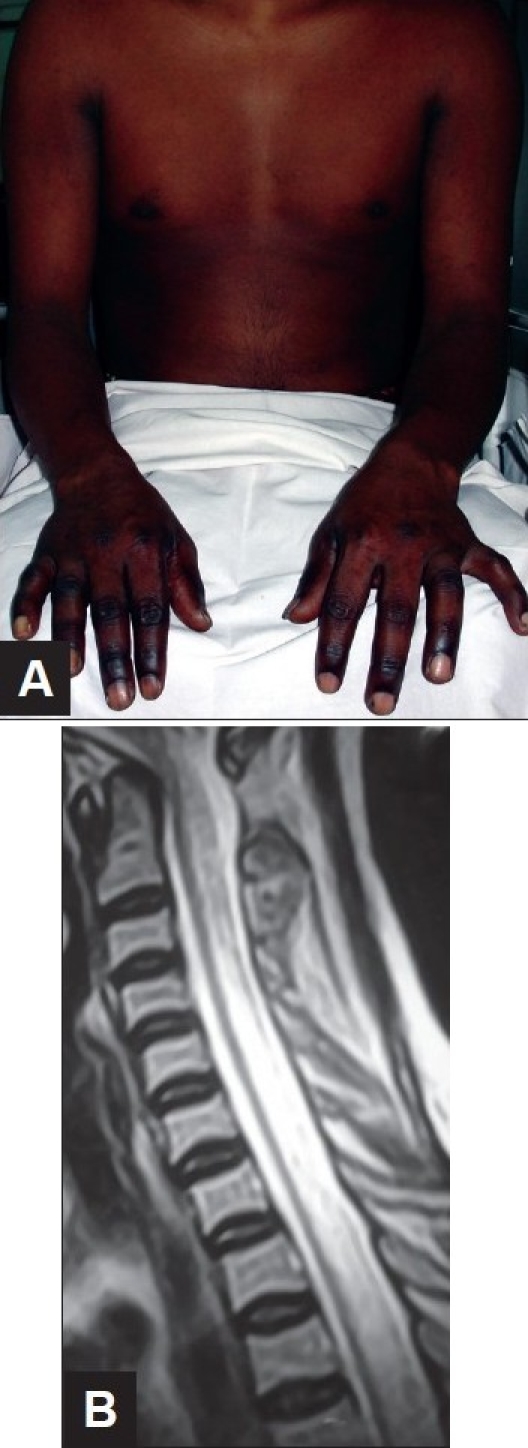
(A and B) 18 year old male was admitted with progressive parapalegia of 3 months duration. Earlier in the year he had similar weakness which improved partially when he was admitted to a local hospital for fever and upper respiratory infection (review of old prescriptions revealed that he had received vitamin supplements). Clinically he was anemic and had hyper pigmentation of distal extremities (Fig. 5A). Peripheral smear and bone marrow confirmed the diagnosis of megaloblastic anemia. MRI of the spinal cord (Fig. 5B) showed on T2W images, linear hyperintense lesion in the cervical and upper dorsal cord sparing the anterior column. He improved moderately with Vitamin B12 therapy
